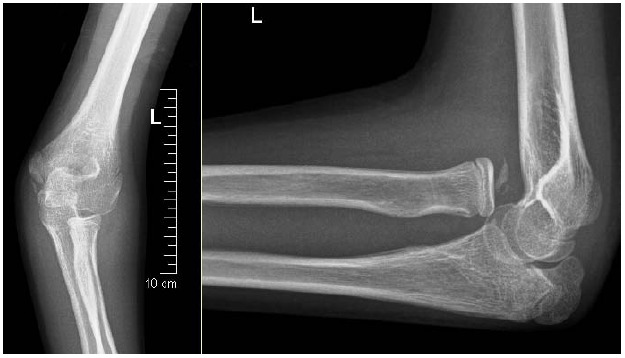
Radiographic picture of a twelve year old girl who sustained injury to her left elbow, showing anterior dislocation of the radial head (Bado type-1 lesion) with calcification of annular ligament remnants.
